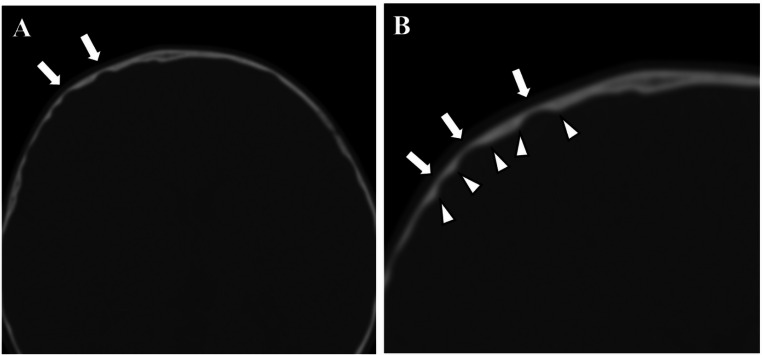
Imaging appearance of CT digital markings. A There are some focal depressions of the inner table in the right side of frontal bone, compared to the contralateral side, defined as CT digital markings (arrows). B Magnified image of right side of frontal bone demonstrates that CT digital markings well demarcated concavity. Note that the concavities exhibit relatively steep peripheral margins (arrowheads) and a smoothly contoured base, where the depth of the focally depressed area is thinner than half of that at the adjacent normal skull bone (arrows)
